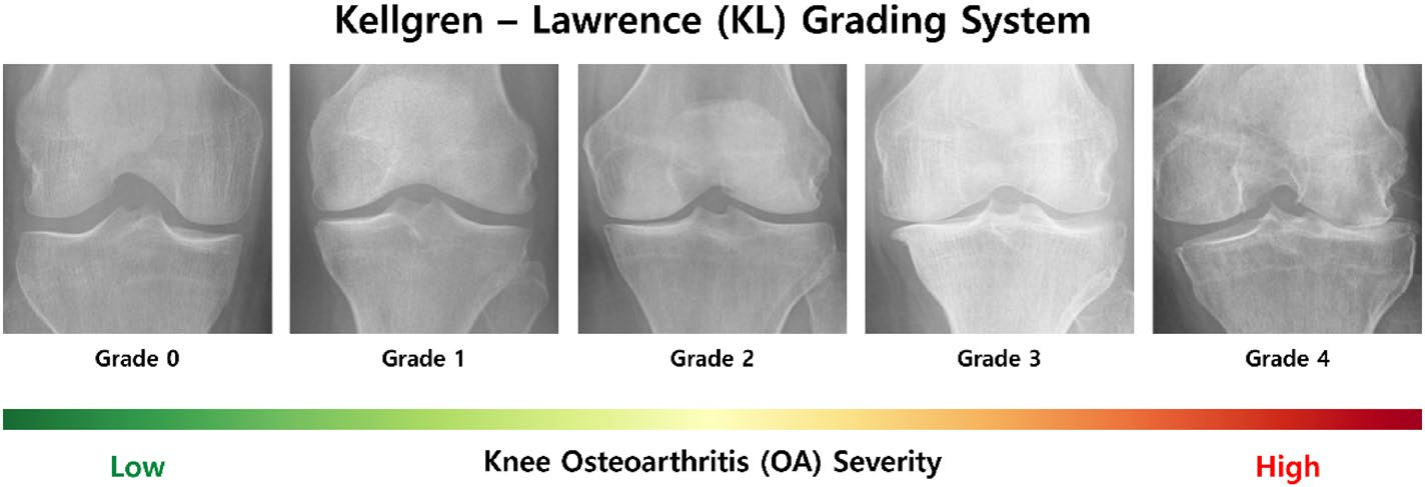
Examples of knee joint X-ray images for each KL grade according to OA severity. The KL grade system categorizes grades according to two crucial aspects of OA: the extent of joint space narrowing and the degree of osteophyte formation.
